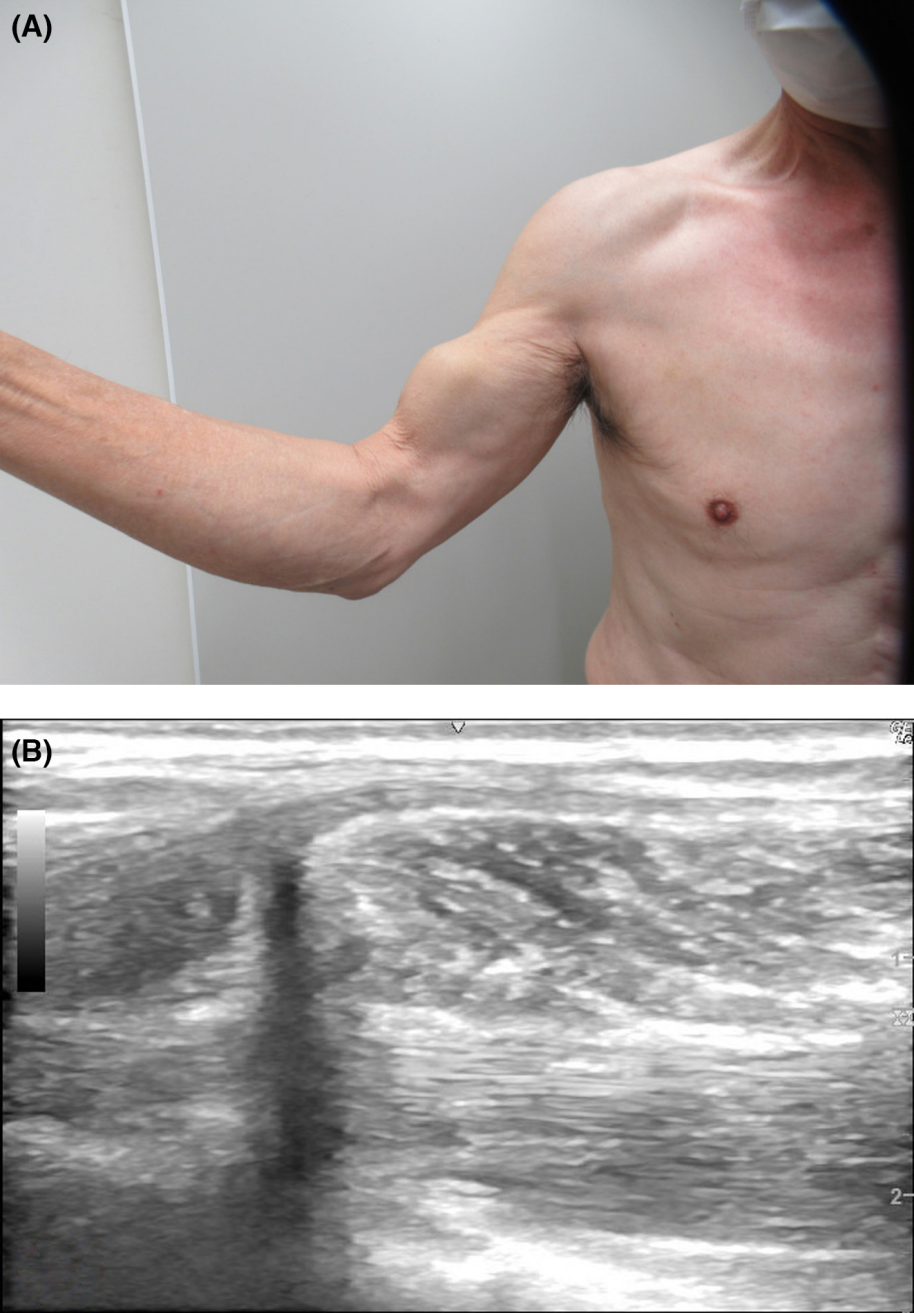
(A): Right upper arm with an obvious bulging appearance. (B): Ultrasonography findings showing no hematoma or tumor. The bulging biceps muscle is shown separating from the humeral articulation and not moving with arm curl or stretch.
